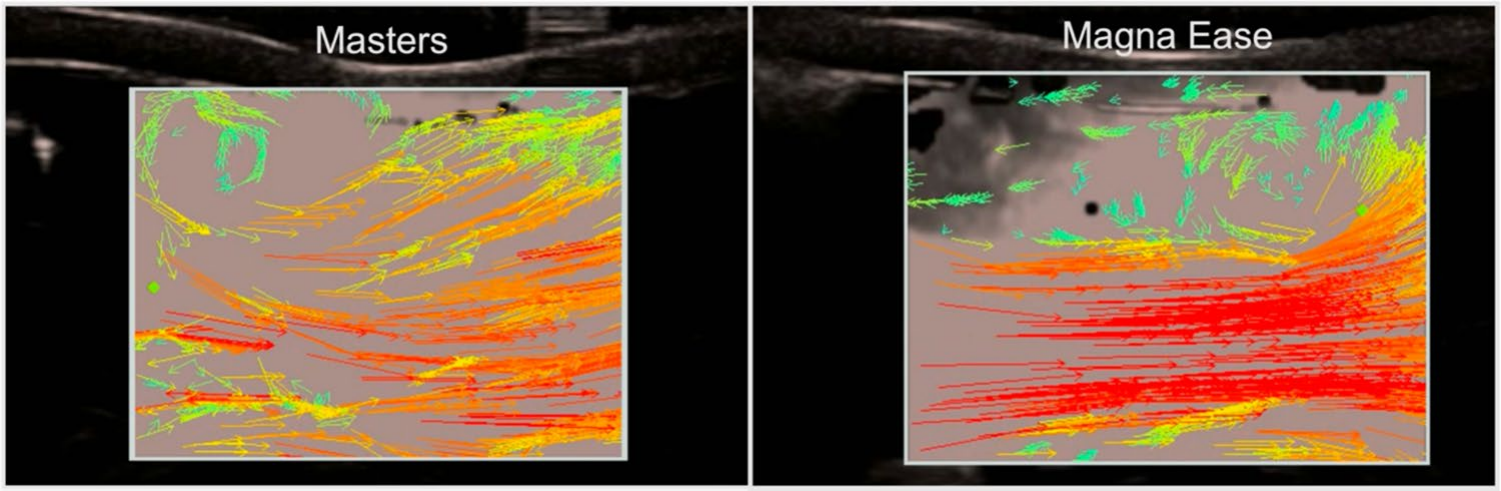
Vector ultrasound of the aortic root for a mechanical (left) and biological (right) valve during peak systole; The measurement plane corresponds to plane 1 from Fig. 7; In both images the vortices at the upper wall are prominently visible with a main difference being the strongly developed central jet in the biological valve, while the jet for the mechanical valve appears more diffuse
